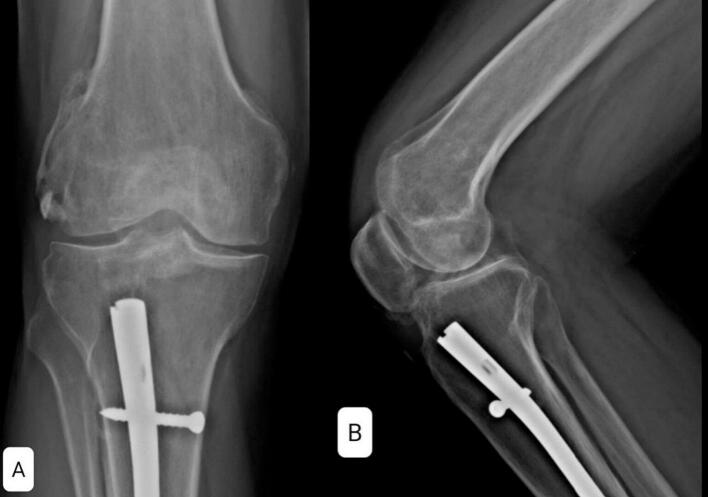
Knee radiography after two years shows heterotopic ossification in the lateral collateral ligament and retropatellar region, with some degree of patella baja. A: AP view B: Lateral view.
